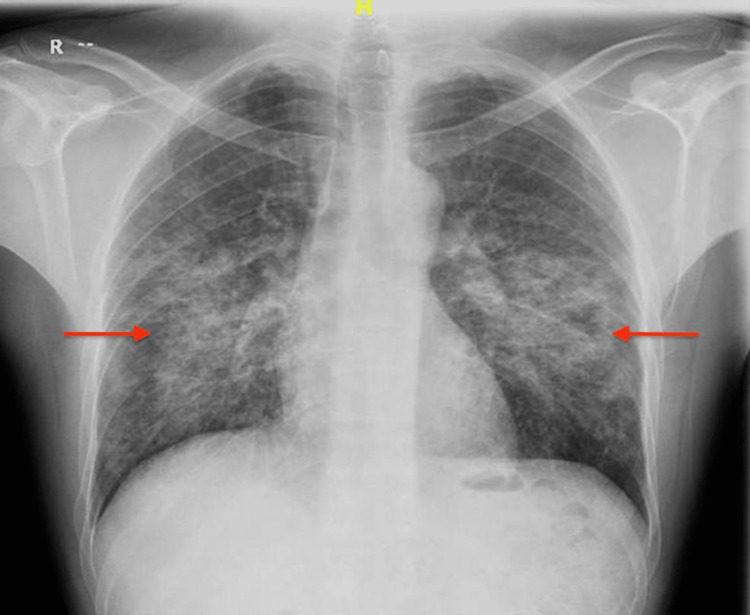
Chest X-ray Bilateral heterogeneous opacities and clear costophrenic angles.
